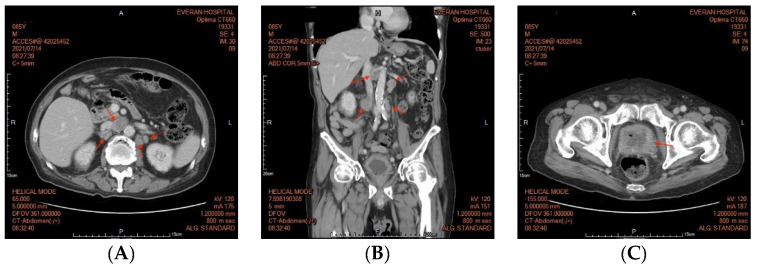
(A) Abdominal computed tomography (CT) revealed multiple lymph node enlargement at the paraaortic area (arrowhead). (B) Sagittal view of the abdomen CT. (C) Abdominal CT of the pelvis revealed a prostate gland with heterogenous density.
